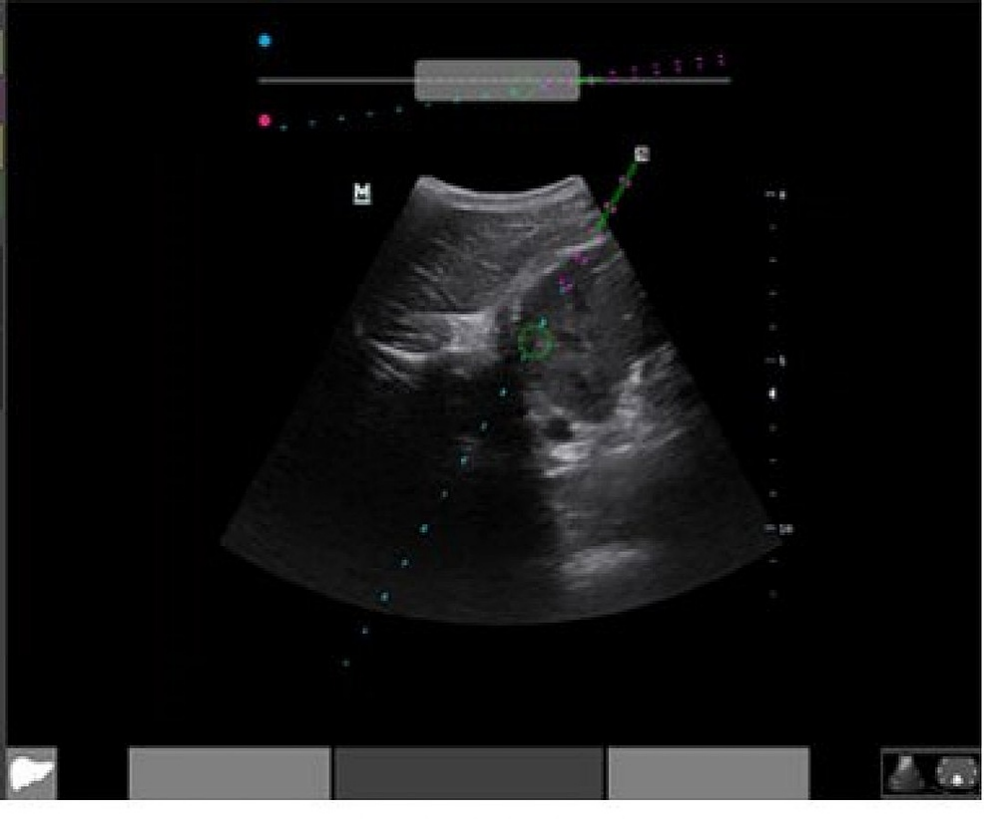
Exemplar ultrasound needle path projection with the Clear Guide system.
